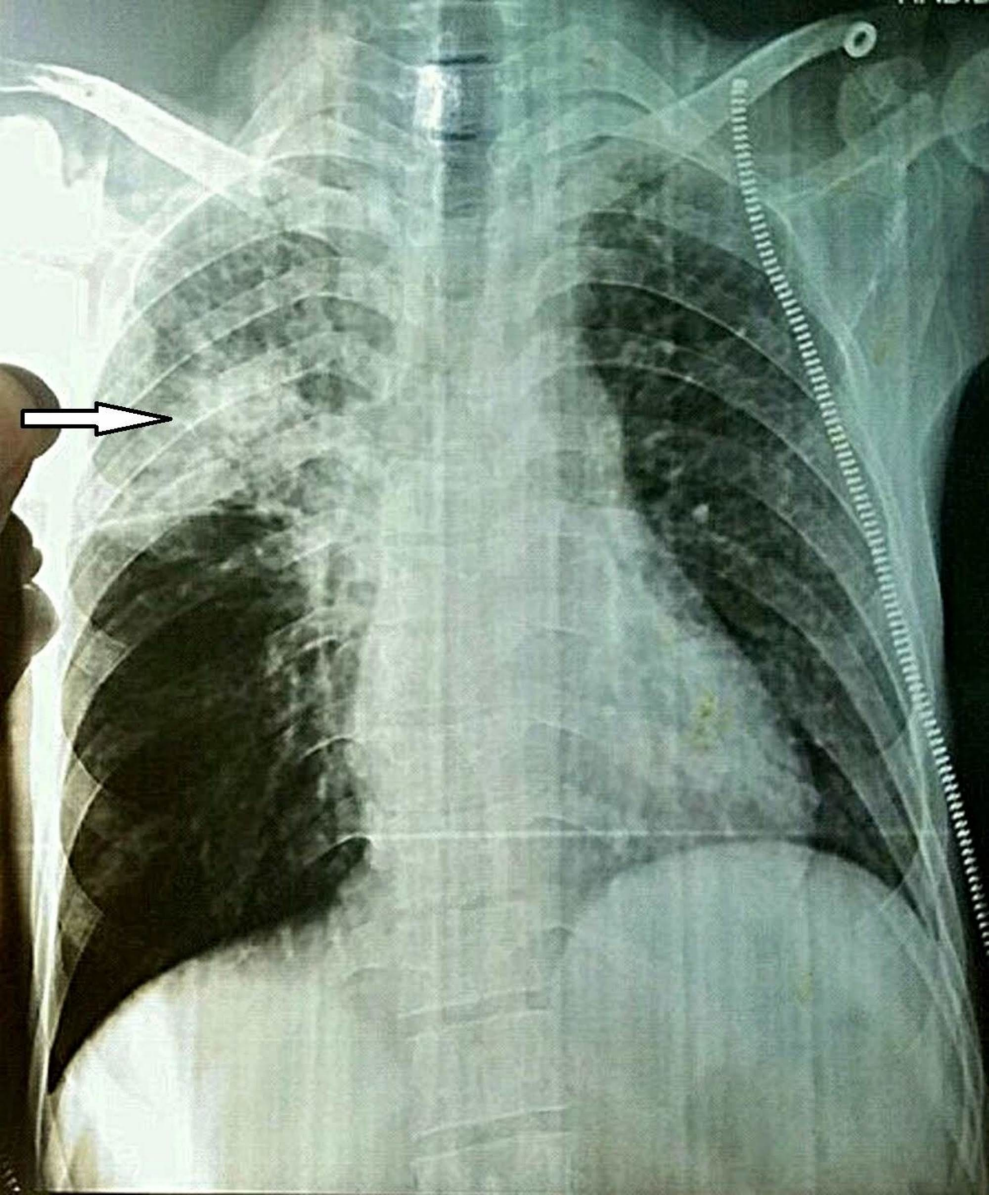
Chest X-ray shows boot-shaped heart with cavitation and fibrosis in the right upper lobe (arrowhead)
